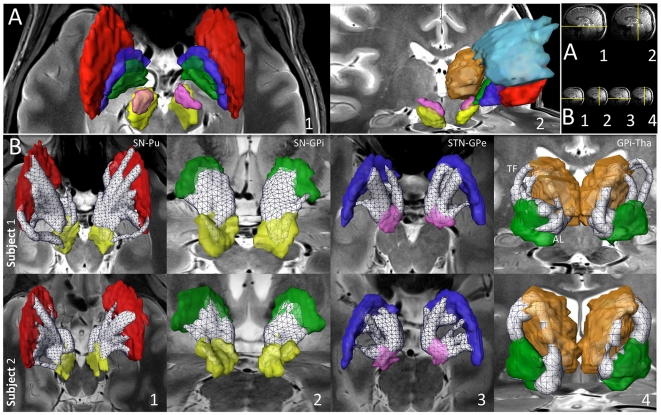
Segmentation and reconstruction of fiber pathways of the basal ganglia and thalamus. (Panel A) Three-dimensional visualization of manual segmentations of basal ganglia and thalamus from high-resolution SWI, T2-weighted images and fractional anisotropy maps. Segmentations are superimposed on T2-weighted images. (Panel B) White matter pathways identified using diffusion MRI probabilistic tractography. The white wireframe volumes represent white matter tracts identified as pathways of interest (see Materials and Methods). From left to right: (1) nigrostriatal, (2) nigropallidal, (3) subthalamopallidal and (4) pallidothalamic pathways with AL: *ansa lenticularis* and TF: *thalamic fasciculus*. Background images are 7T T2-weighted MR images. (Top right inset) Yellow lines depict the orientation and location of T2-weighted images in each panel. (A1) Axial image at the level of SN; (A2) Coronal image at the level of the posterior Tha; (B1) Axial image at the level of SN, caudo-rostral orientation; (B2) Coronal image at the level of the anterior GPi; (B3) Axial image slightly inferior to STN to avoid obscuring portions of the tract; (B4) Coronal image at the level of the posterior Tha, rostro-caudal orientation. Color code: Caudate nucleus, CN: light blue; Putamen, Pu: red; External globus pallidus, GPe: dark blue; Internal globus pallidus, GPi: green; Substantia nigra, SN: yellow; subthalamic nucleus, STN: magenta; Thalamus, Tha: orange.
